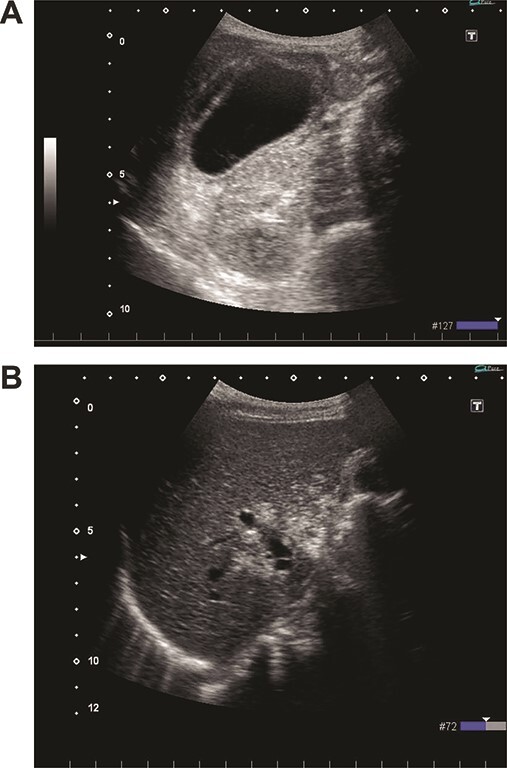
(A) Ultrasonography shows distention and wall thickening of the gallbladder. (B) Ultrasonography shows small mobile echogenic foci and hyperechogenic linear structures in the middle segment and right anterior segment of the liver.
